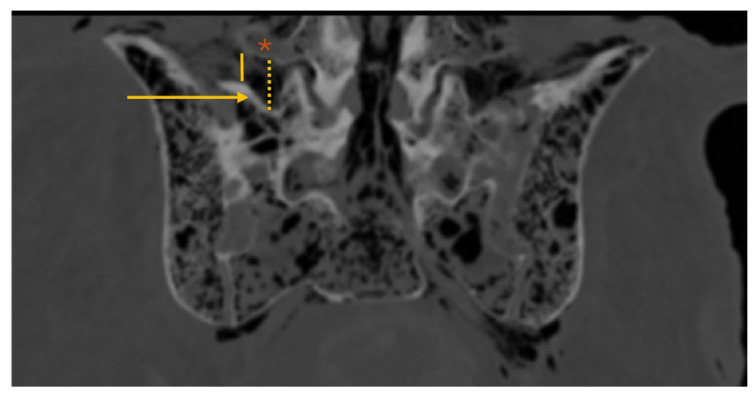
Lumbosacral spine CT—coronal plane; *: L5 transverse process (TP); arrow: sacral ala; dotted yellow line: maxL5TP–sacrum (maximum distance from the inferior border of the L5 TP to the superior border of the sacral ala); solid yellow line: minL5TP–sacrum (minimum distance from the inferior border of the L5 TP to the superior border of the sacral ala).
